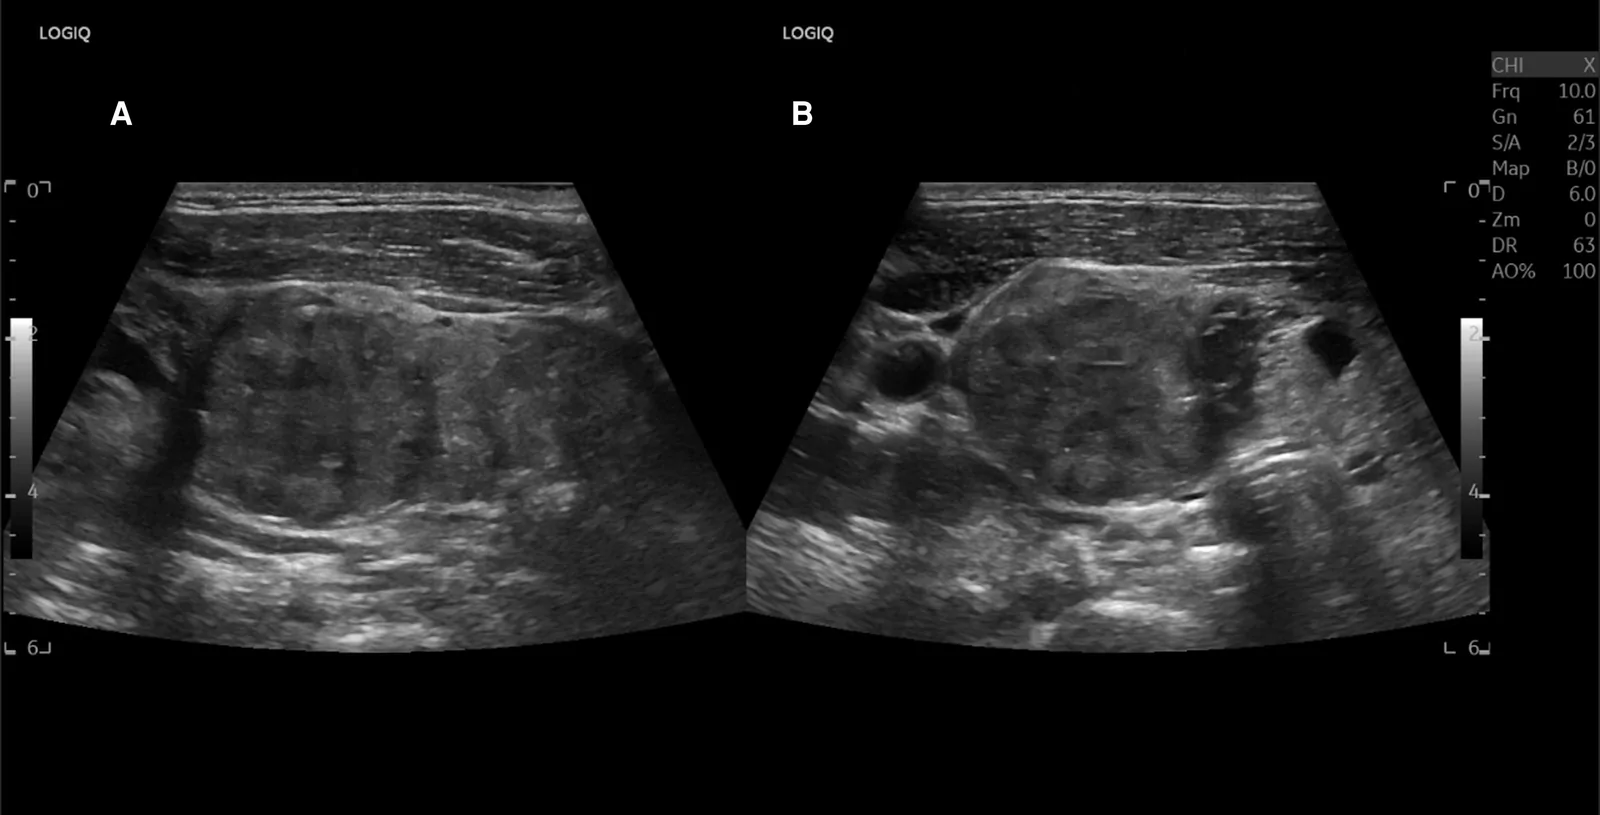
Ultrasound of the thyroid. (A) Sagittal view of the right lobe. (B) Sagittal view of the left lobe. Source: JC.
